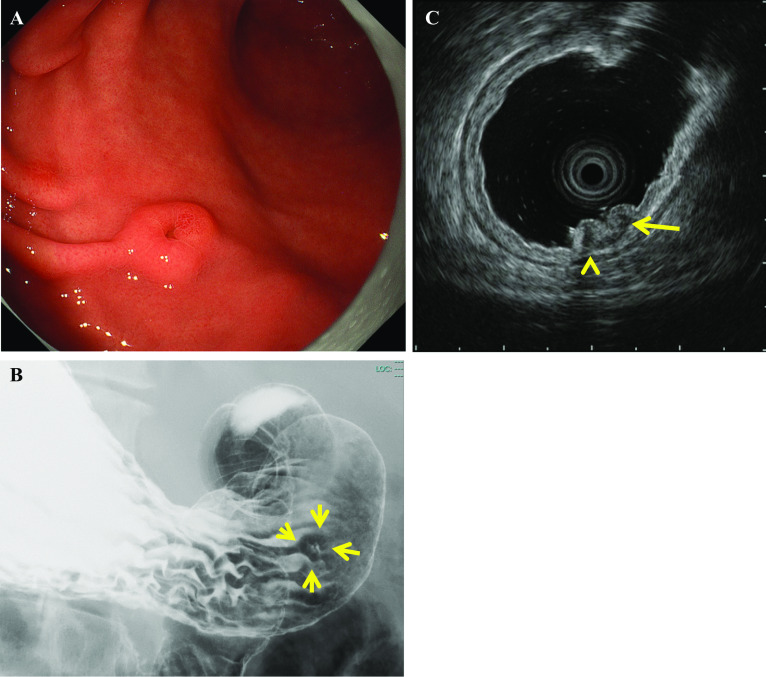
A Esophagogastroduodenoscopy findings during the preoperative examination for gastric submucosal tumors. An elevated lesion, 8 mm in size, with depression in the center, covered with normal mucosa was observed in the anterior wall of the antrum. B Upper gastrointestinal series findings. A raised lesion with a central depression, 8 mm in size, was found in the greater curvature of the gastric body (arrow). C Endoscopic ultrasonography findings. A 10-mm tumor was found in the second layer (arrow). Because of the thinning of the third layer, submucosal invasion could not be completely ruled out (arrow head)
